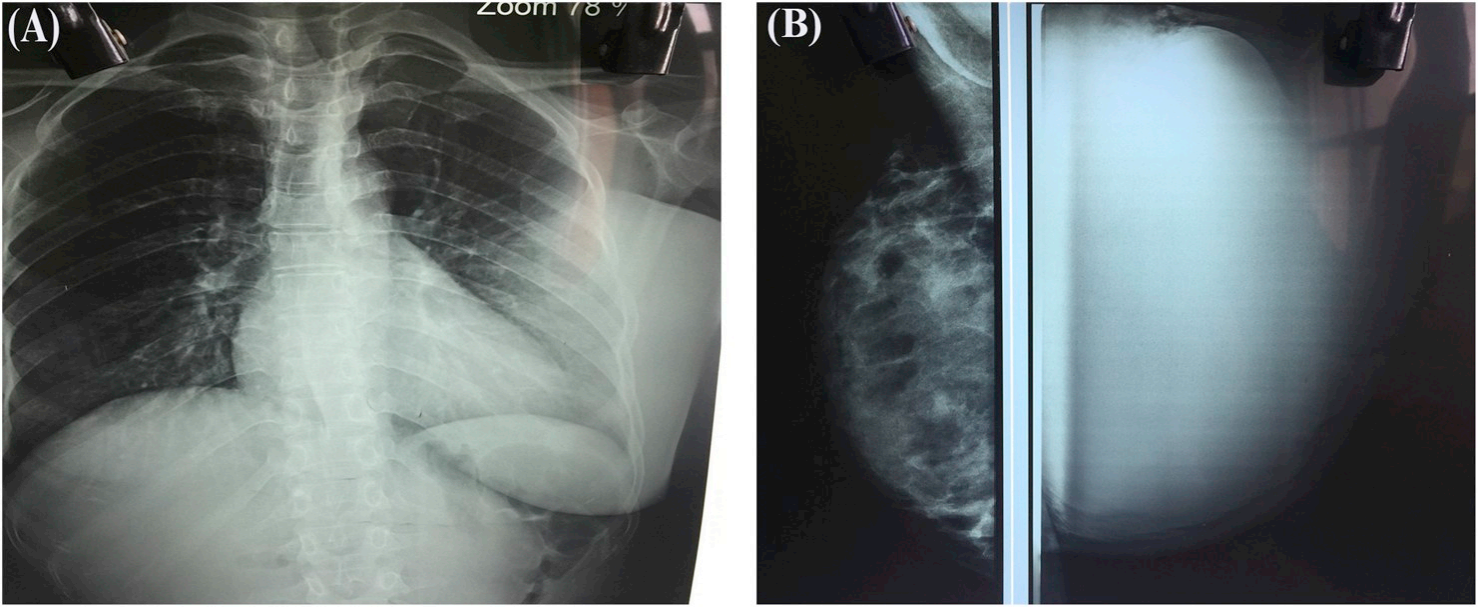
(a) Chest X-ray showing prominent breast shadow on left (b) Radiodense lobulated mass in mammogram without calcifications.
